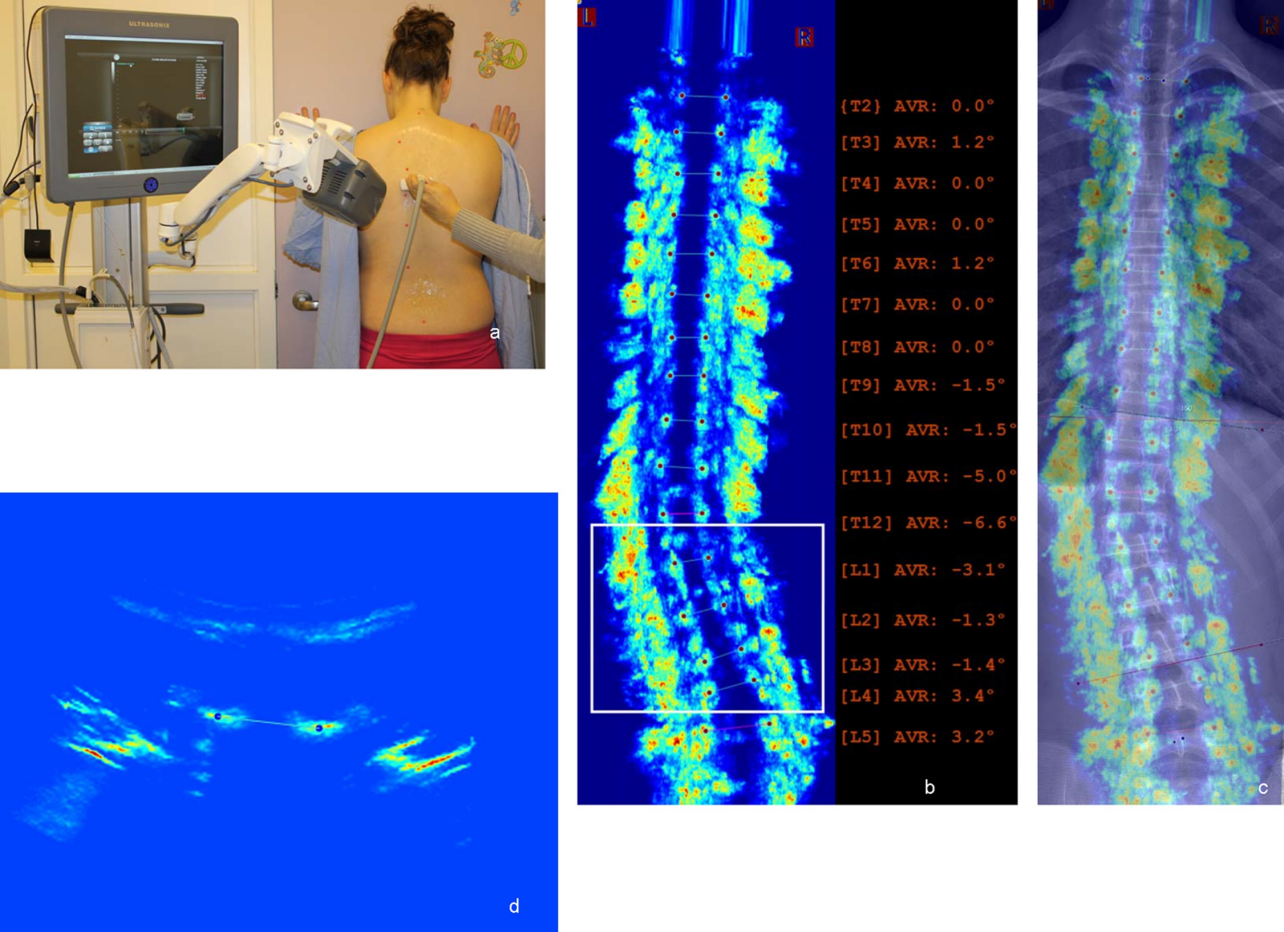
The US measurement on a scoliotic subject. (a) the subject being scanned using SonixTABLET medical ultrasound system, (b) the US coronal image with the measurements of vertebral rotation, (c) the overlaid US coronal image on the previous radiograph, and (d) the US transverse image at apical level (T12).
